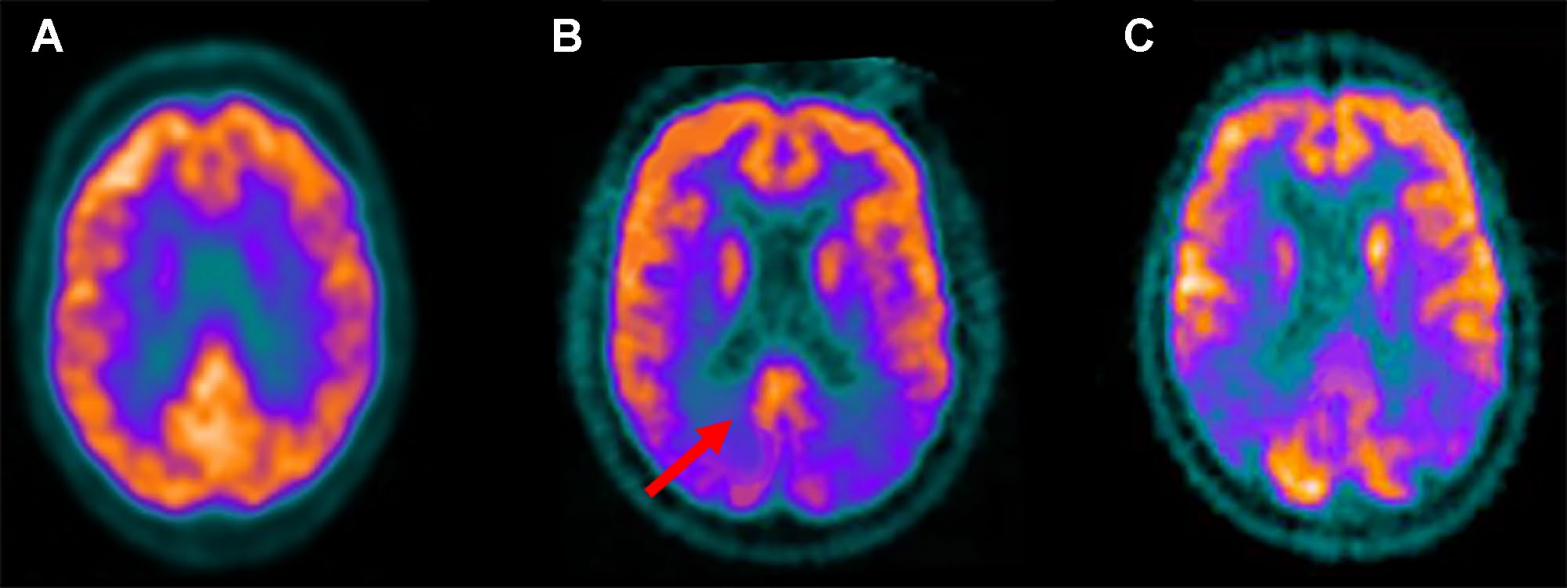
Fludeoxyglucose F 18 (18F-FDG) positron emission tomography standard axial view transacting the posterior cingulate region obtained in a healthy control (A); in a patient with Lewy bodies dementia (DLB) (B); in an Alzheimer disease (AD) patient (C). Note that reduced 18F-FDG uptake, indicative of glucose hypometabolism and reduced synaptic activity, is present in the occipital lobes while preservation of metabolic activity is found in the posterior cingulate cortex (red arrow). This feature, known as the “cingulate island sign,” is often seen in DLB but not AD patients.
